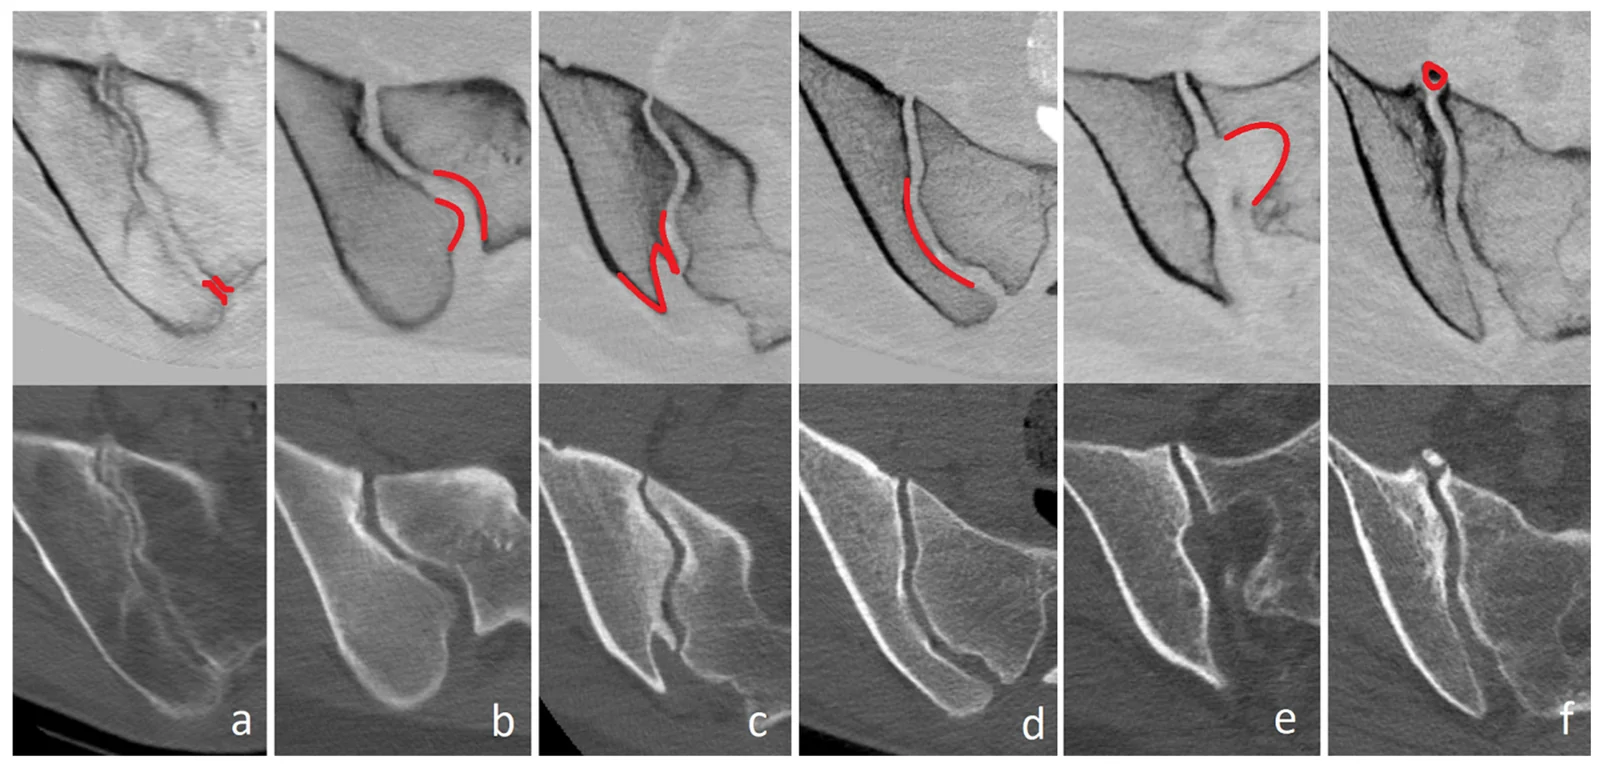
Coronal sacroiliac computed tomography sections and their inverted versions, the latter used to improve conspicuity of the cortical outlines, demonstrate the anatomic variants of the sacroiliac joints: accessory sacroiliac joint (a), iliosacral complex (b), bipartite iliac bony plate (c), crescent iliac bony plate (d), semicircular defect (e), and unfused ossification center (f). Red markings on the inverted images outline the variant anatomy.
